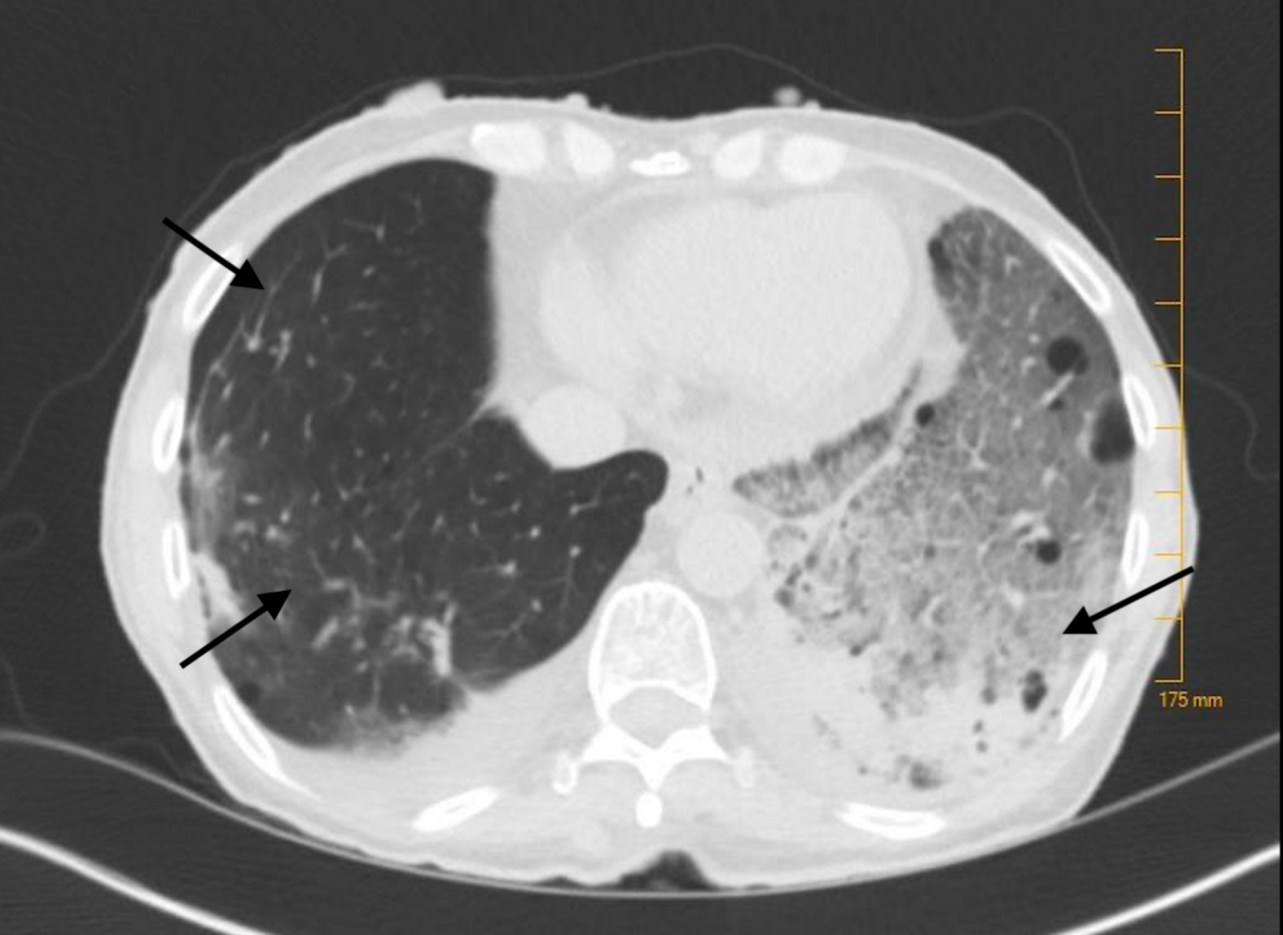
CT chest showing multiple cysts and left basilar consolidation with ground glass opacities.
